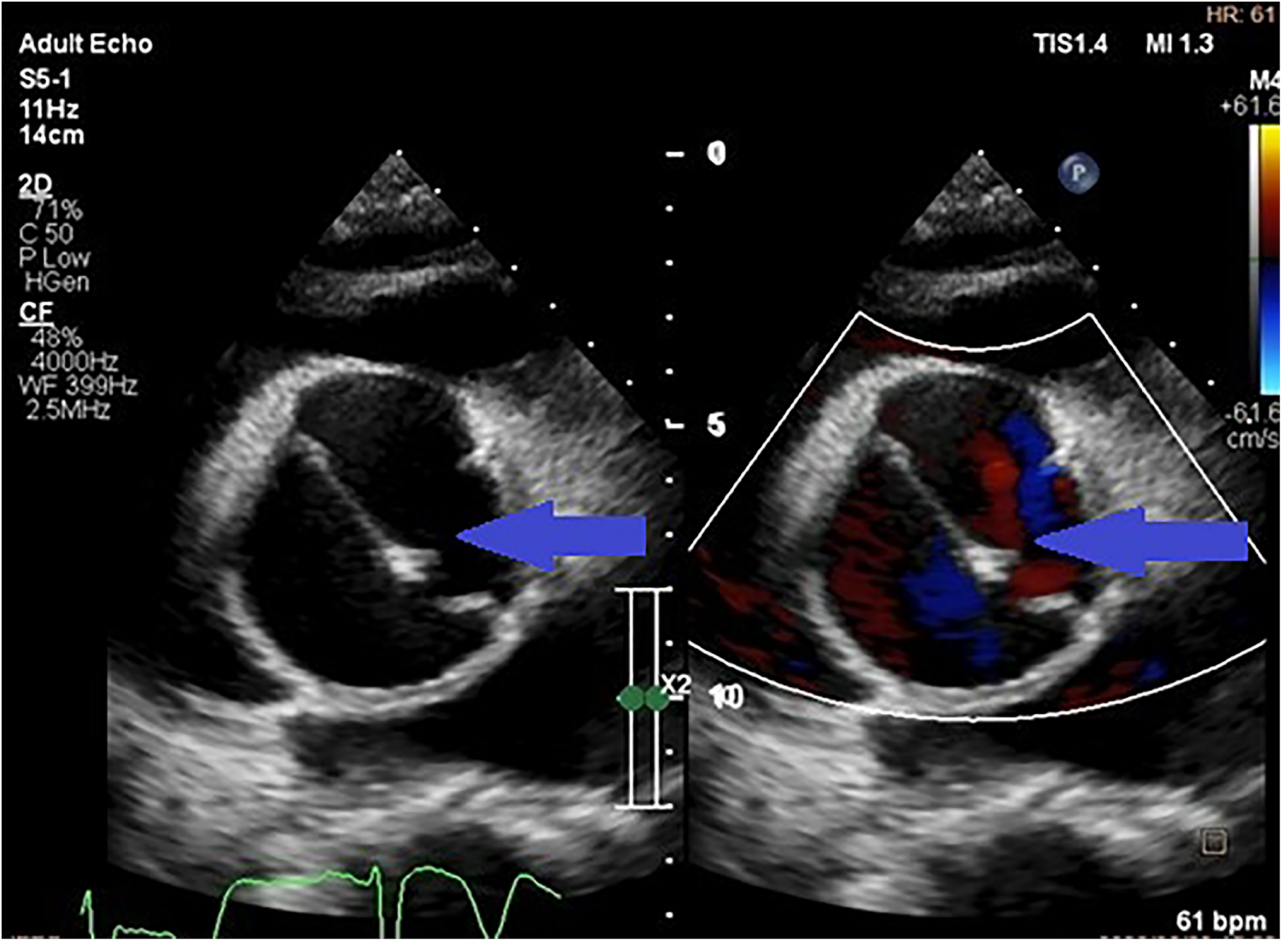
Parasternal short axis transthoracic view showing a dissection flap in an aneurysmal thoracic aorta (indicated by the blue arrows) in a patient with uncontrolled hypertension.
